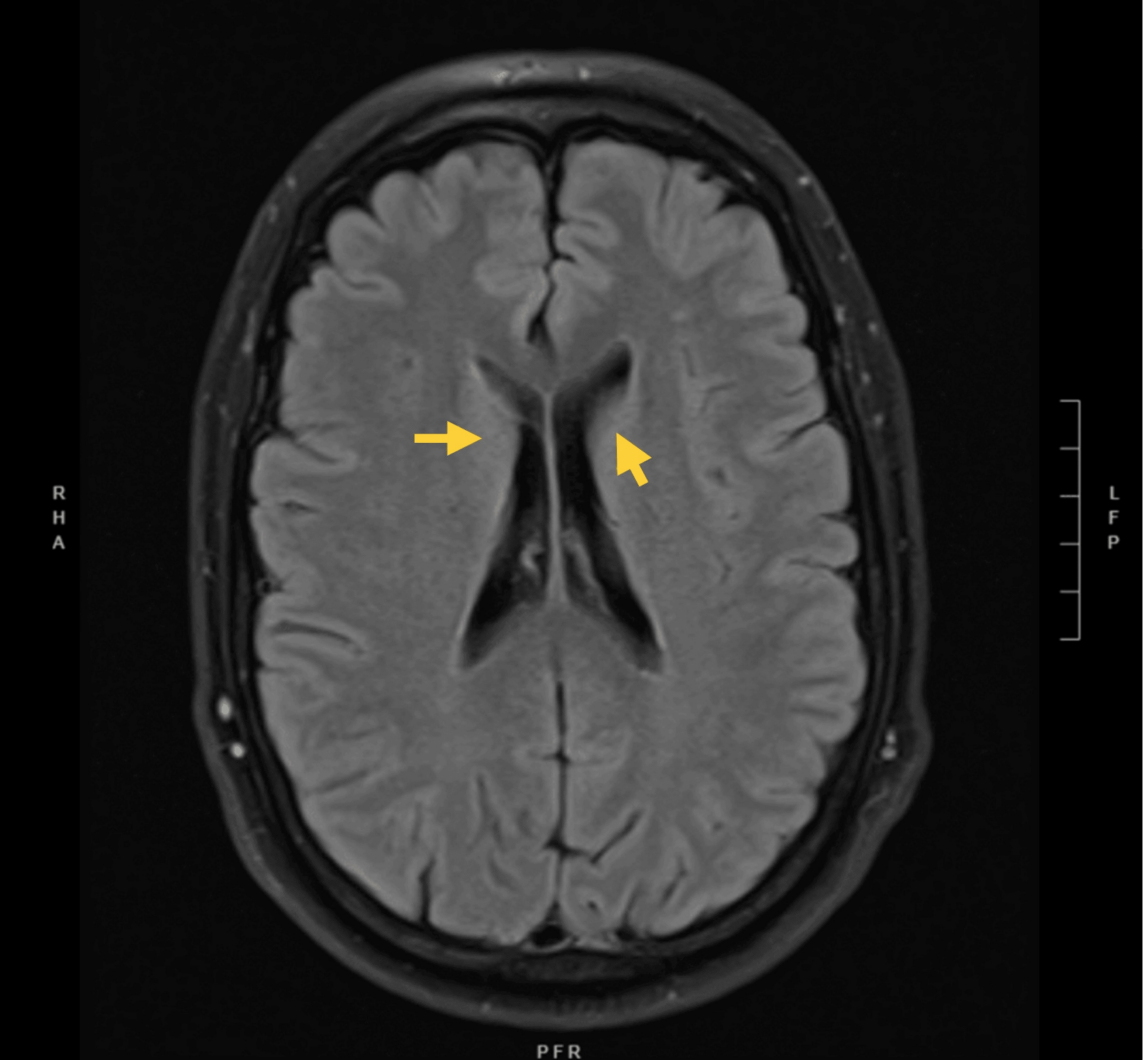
T2 fluid attenuated inversion recovery (FLAIR) MRI sequence showing several small white matter FLAIR hyperintensities around ventricles likely secondary to chronic small vessel ischemic disease (yellow arrows).
